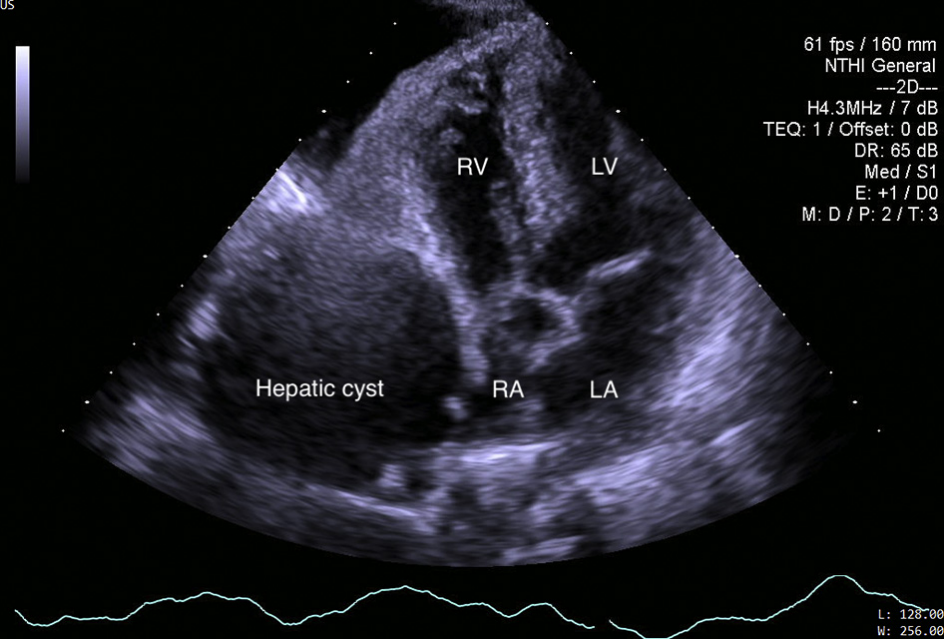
The hepatic cyst compressing the right atrium seen on transthoracic echocardiography.
